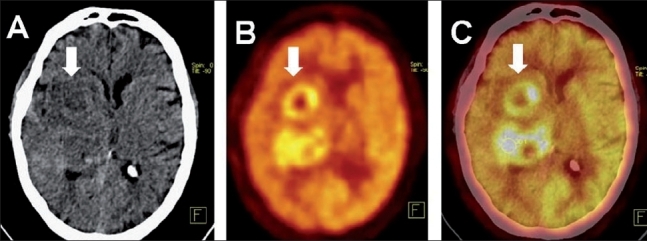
Cerebral tuberculous abscess. A 70-year-old diabetic with acute-onset hemiparesis. Axial plain CT (A), PET (B), and PET/CT (C) images show hypodense lesions in the right basal ganglia and thalamus (arrows) with a mass effect. PET shows two ′doughnut lesions,′ with peripheral FDG concentration and central cold areas. The pattern is suggestive of, though not specific for, tuberculous abscesses. Stereotactic biopsy revealed tuberculosis
